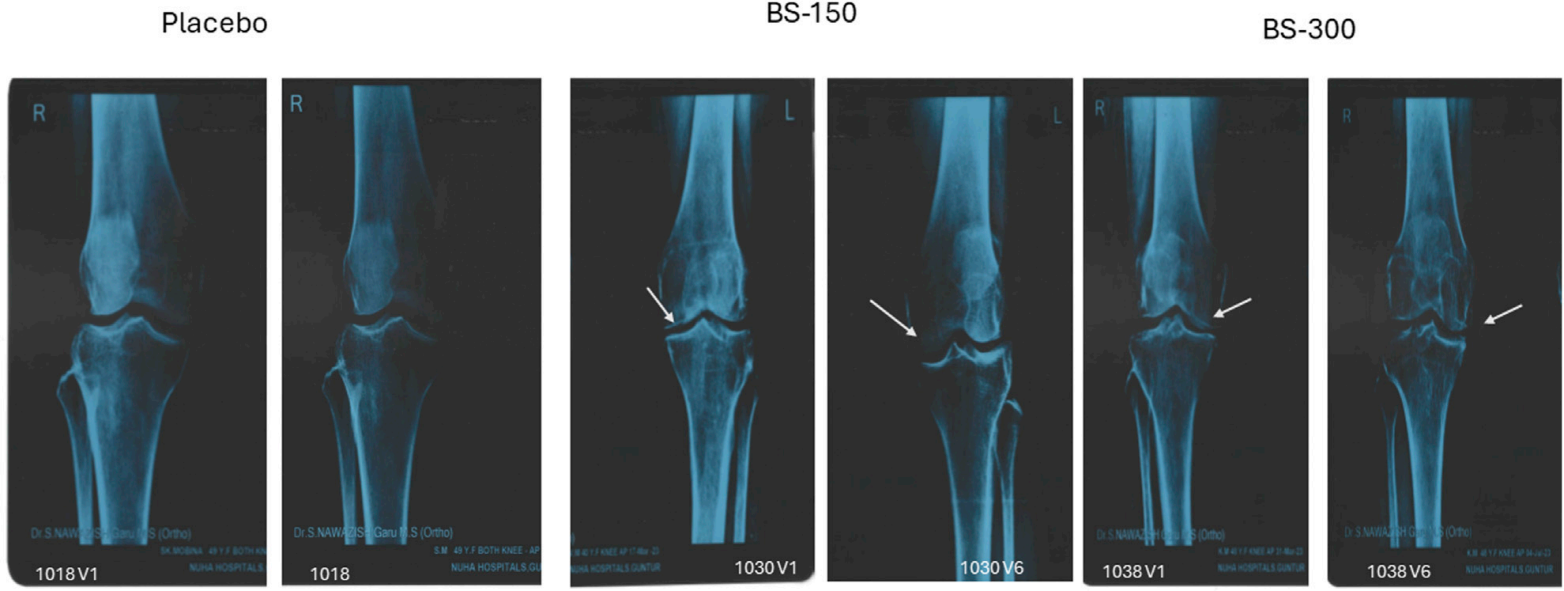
Radiological X-ray image. Radiological X-ray images of the knee of participants captured on screening and end of the study. Significant improvements in osteoarthritis was observed in the knee joint. The gap between the knee joints (white arrow) increased significantly in BSE-150 and BSE-300 supplemented participants.
